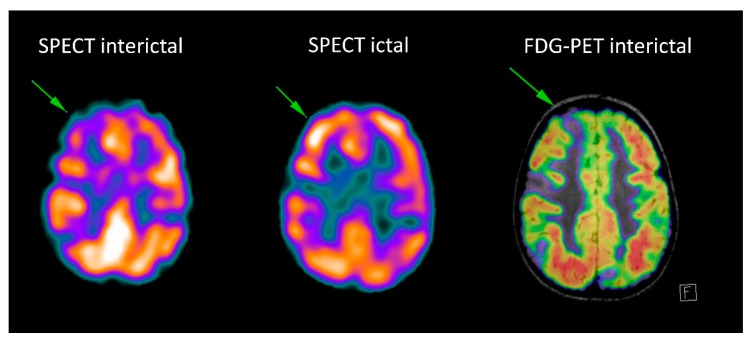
Patient with tuberosis sclerosis complex: the interictal Single-photon emission computed tomography (SPECT) and fluorodeoxyglucose-positron emission tomography (FDG-PET) (fused with MRI FLAIR images) show multiple hypometabolic foci, corresponding to the multiple tubers. Ictal SPECT shows ictal hyperperfusion of the superior right frontal tuber.
